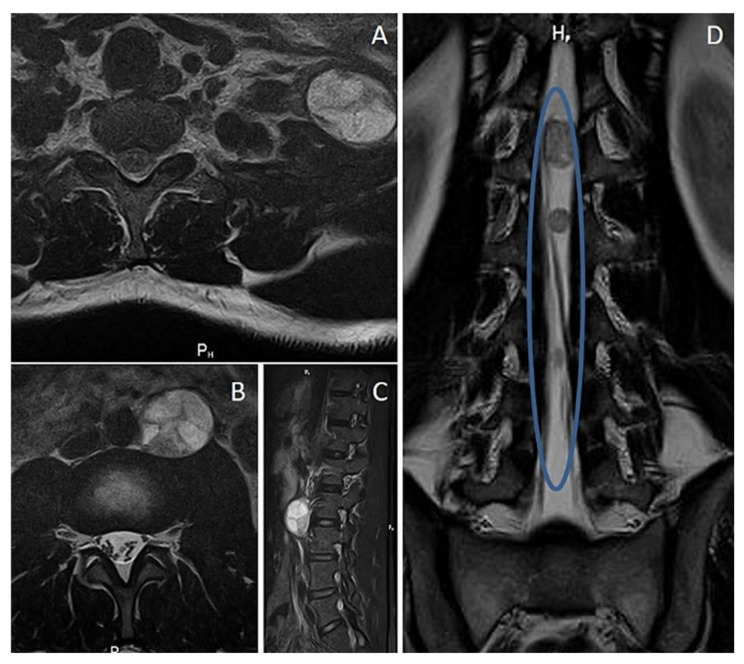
Multiple neurofibromas in an NF1 patient. (A) Left laterocervical mass, non-homogeneous, well-defined T2 axial view; (B) left lumbar para-aortic mass on T2 axial view and (C) STIR sagittal view. (D) Intracanalar lumbar tract myelography MRI.
